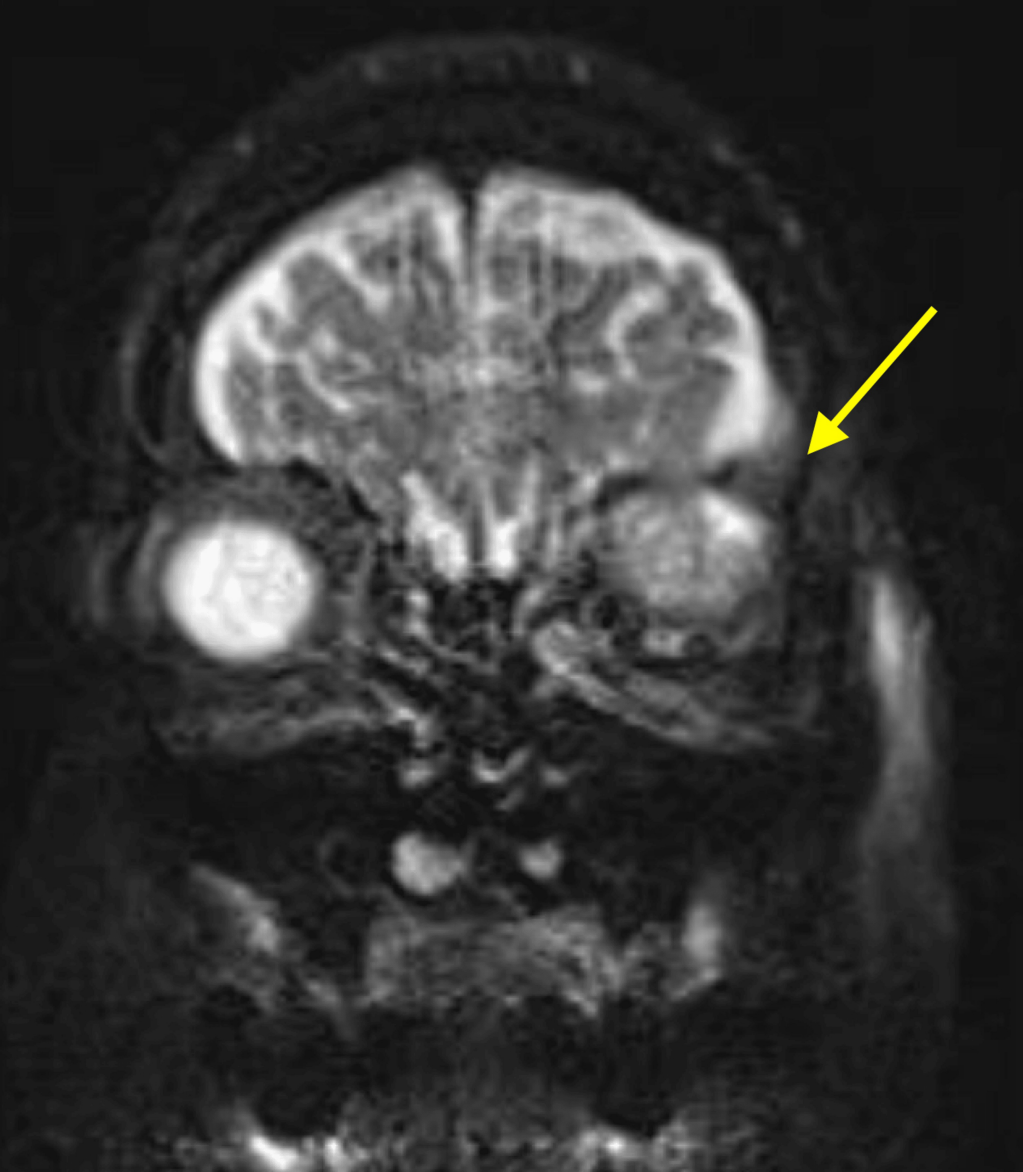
Orbital MRI with and without intravenous contrast showing a large, avidly enhancing, solid and cystic mass in the left superior orbit with intracranial extension through the left orbital roof. There is direct dural involvement and mass effect on the left inferior frontal lobe. The yellow arrow denotes the left orbital mass, which displaces the globe and invades the left orbital roof.
